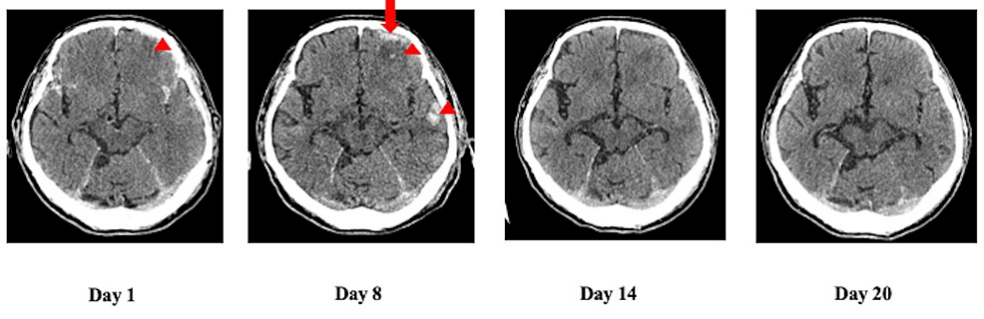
Time course of intracranial lesions Subcutaneous and epidural hematoma in the occipital regions (red arrow) and subdural hematoma in the left frontal lobe (red arrowhead). The CT scan on day 8 revealed a temporary cerebral contusion in the left frontal and temporal lobes, but the CT scan on days 14 and 20 was almost normal.
